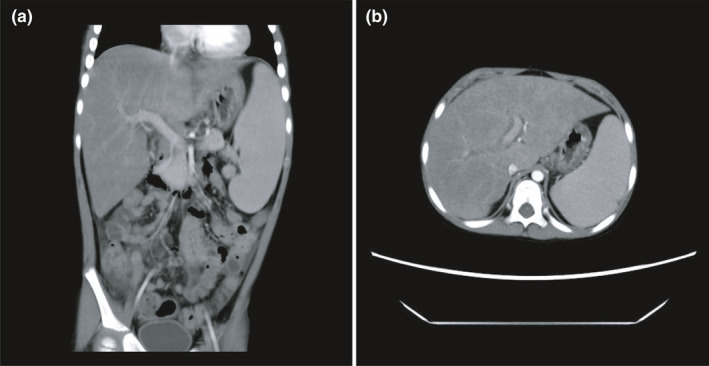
Computed tomography (CT) scan of the upper‐abdomen. The result showed that the patient had both hepatomegaly and splenomegaly. (a) Coronal sections and (b). Axial sections
